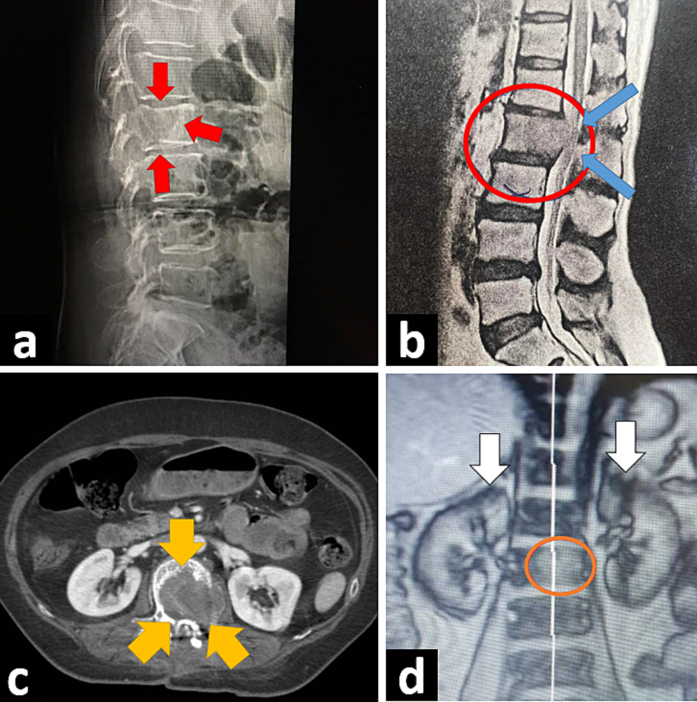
a. Lumbar X-ray (lateral view), showing lytic lesion in second lumbar vertebra (red arrows). b. Lumbar MRI, sagittal view, showing tumor in second lumbar vertebra (red circle) causing cord compression (blue arrows). c. Abdominopelvic CT scan showing vertebral mass (yellow circles) without any kidney tumor. d. Coronal reconstruction of abdominopelvic MRI, showing tumor in vertebra (green circle) and normal kidneys (white arrows). (For interpretation of the references to colour in this figure legend, the reader is referred to the web version of this article.)
